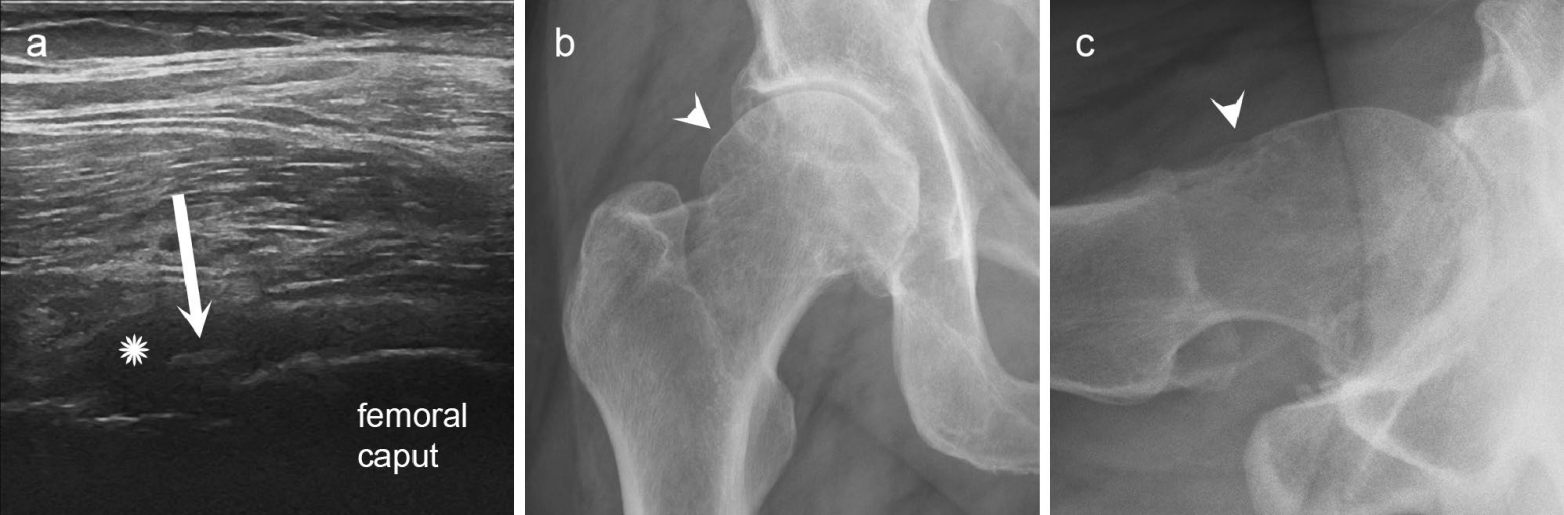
An example case where ultrasonography (US) detected osteophyte and radiography did not. In longitudinal US view of the hip joint (a), a large femoral collum osteophyte is observed (white arrow), whereas on anteroposterior (b) and lateral conventional radiographic views (c) only doubtful bony prominence but no definite osteophytes are seen (white arrowheads). Additionally, the US demonstrates an effusion (white asterisk) of the hip joint.
